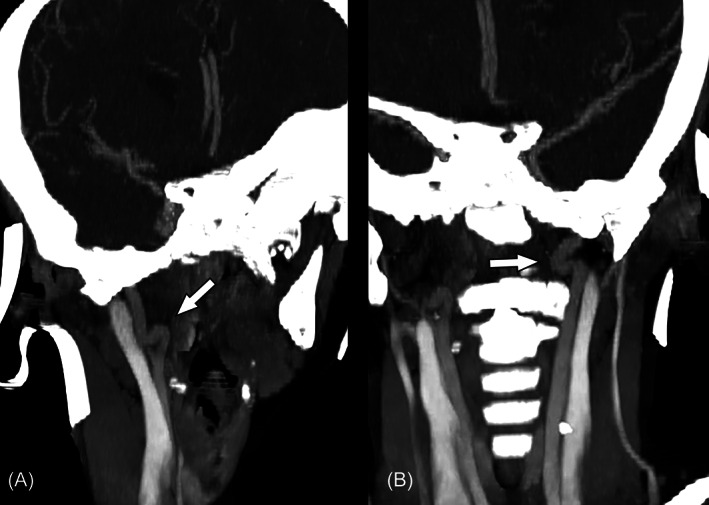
CT‐angiography images (A and B) show tortuosity of both distal portions of cervical internal carotid arteries (arrows)
